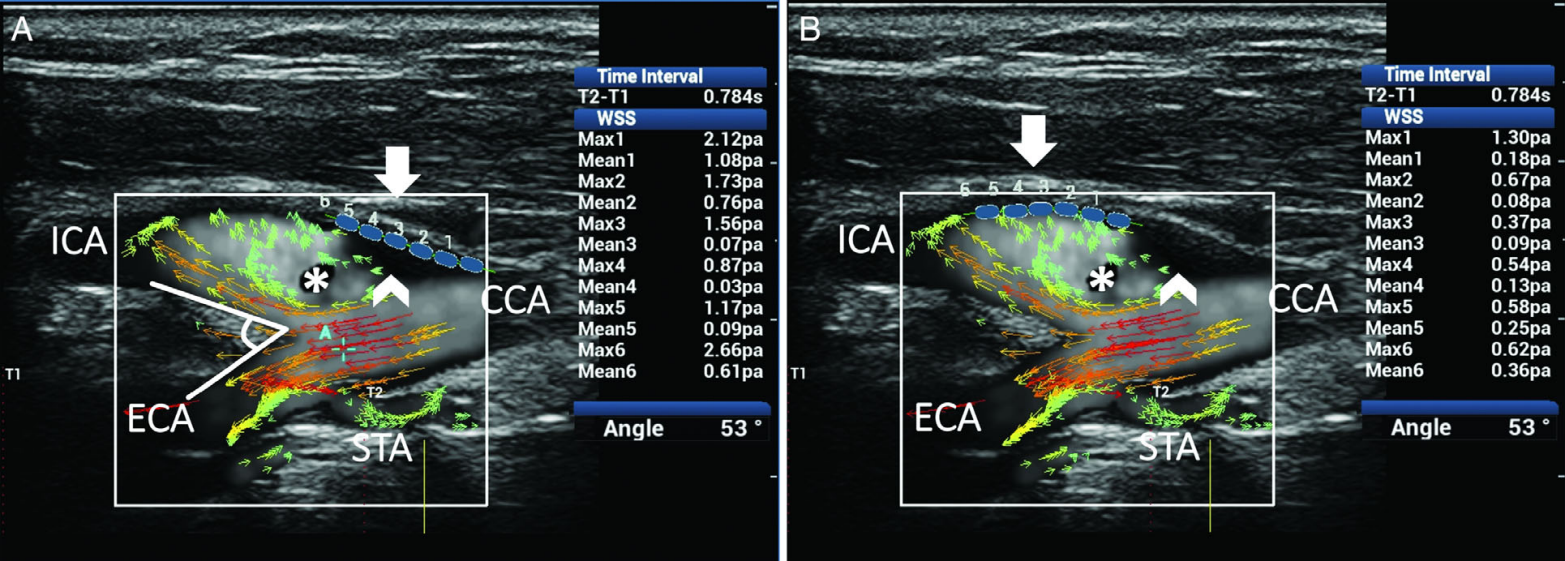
Complex flow in a CB with a 53° bifurcation angle. Ultrasound vector flow imaging shows the development of complex flow due to vessel geometry and bulb–internal carotid artery (ICA) sinus dilatation. High‐velocity red vectors flowing near the divider in the ICA and a wide area of recirculation with low‐velocity green vectors moving in a counter eddy (asterisk) are shown in the outer side of the vessel. Areas in the lumen with no vector arrows and with no background speckle (asterisk and arrowhead) correspond to a flow so slow (near 0 velocity) that it cannot be detected by the system in this specific image or are not displayed because of the setting value of gain selected by the operator. Two series of 6 WSS measurements (A and B) on the outer wall (blue dots) of the bulb and ICA both gave abnormal maximum values (<1.0 Pa; range, 0.37–0.87 Pa) in 6 of 12 sample points and mean values (<0.4 Pa; range, 0.03–0.36 Pa) in 9 of 12 sample points. CCA indicates common carotid artery; ECA, external carotid artery; and STA, superior thyroid artery.
